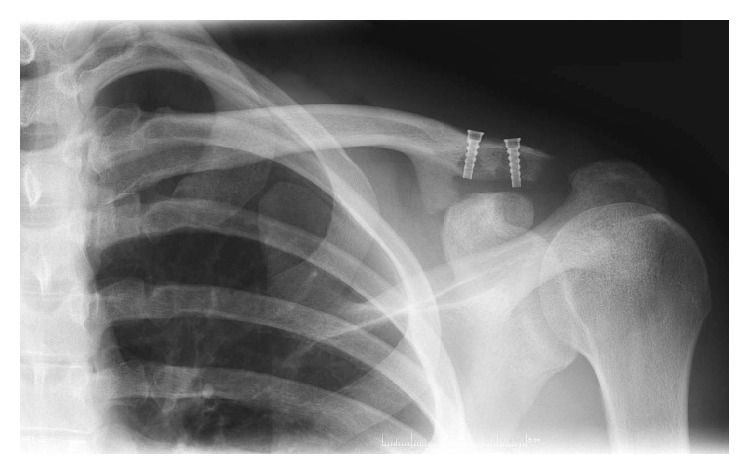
Anteroposterior left ACJ radiograph two months after re-do LARS implantation showing anatomically reduced ACJ.
